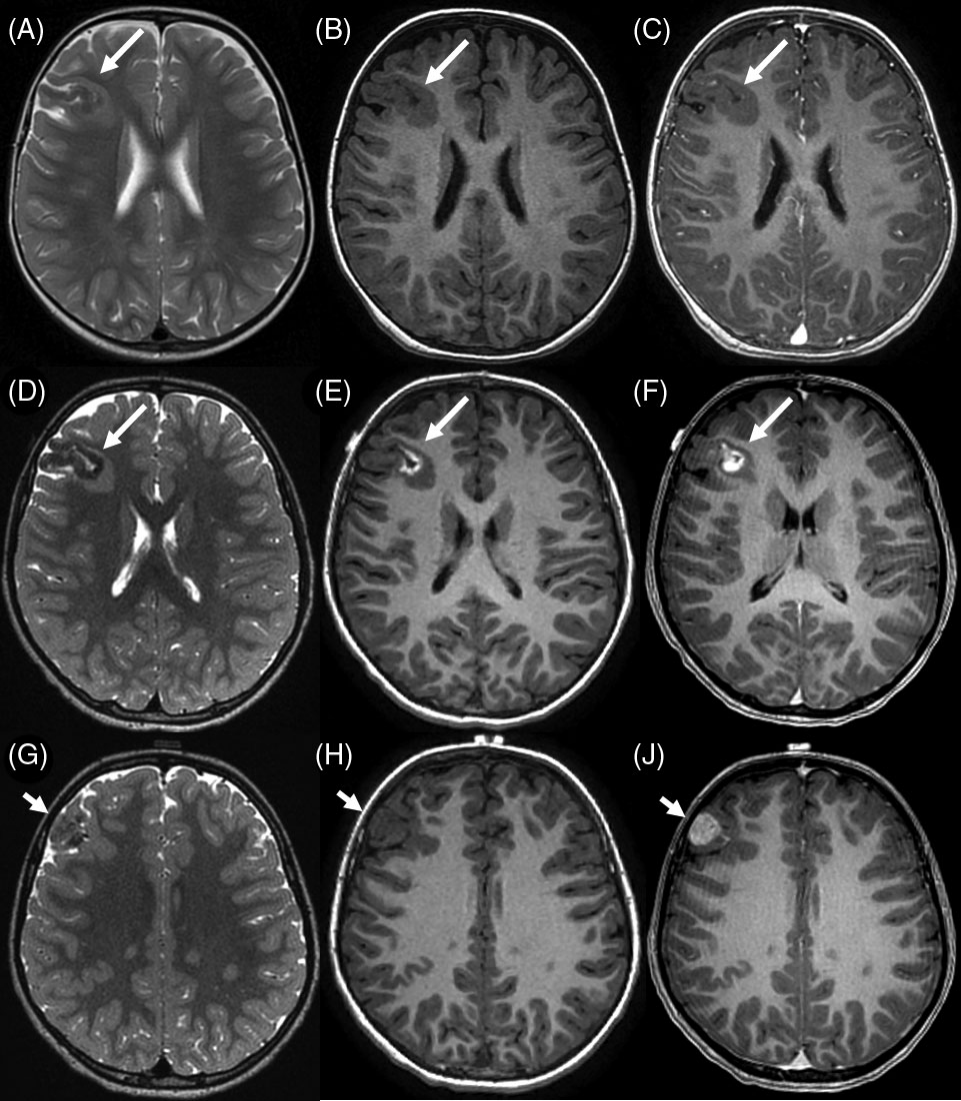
Neuroradiological features of Case #9. Magnetic Resonance Imaging performed at diagnosis at the age of 2 (A)–(C) and before surgery at the age of 7 (D), (I). The right frontal meningioangiomatosis is observed as cortical thickening with T2‐weighted low intensity signal (A), (D), an intermediate signal on T1‐weighted images at diagnosis (B), but high intensity signal during follow‐up (D), consistent with calcification on computed tomography (not shown), and very small contrast enhancement (F). Additionally, a small subcortical T2‐weighted high intensity signal is present (D). An extra‐axial mass with T2 and T1‐weighted low intensity signal (G), (H) and strong contrast enhancement (I) is indicative of an associated meningioma. It was not visible on the initial Magnetic Resonance Imaging scan (not shown).
